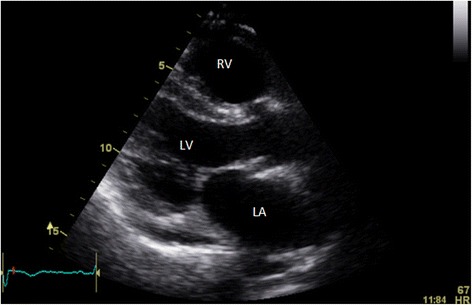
Parasternal long view in systole (2 weeks post pericardiocentesis and development of LV dysfunction), showing resolution of both akinesis in the mid septum and apical ballooning (apex not well visulised here)
